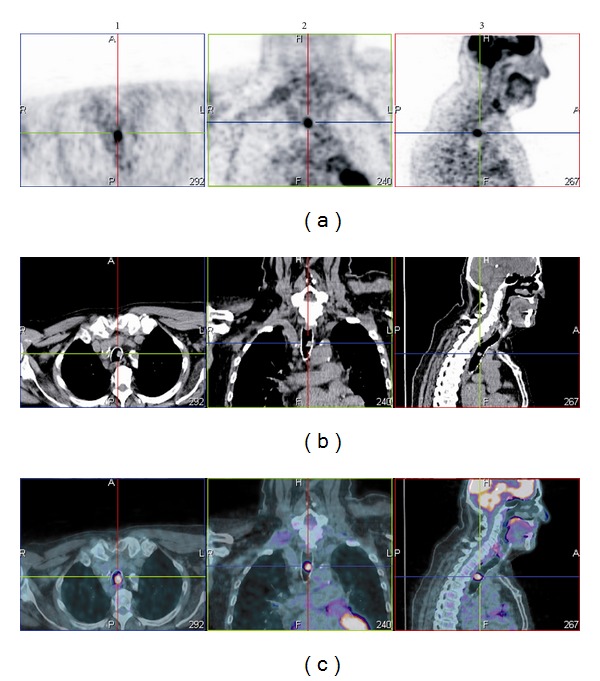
PET scan shows a localized FDG uptake in the middle third of the trachea. (a): PET, (b): CT, (c): PET-CT. (1): axial view, (2): coronal, (3): sagittal.
